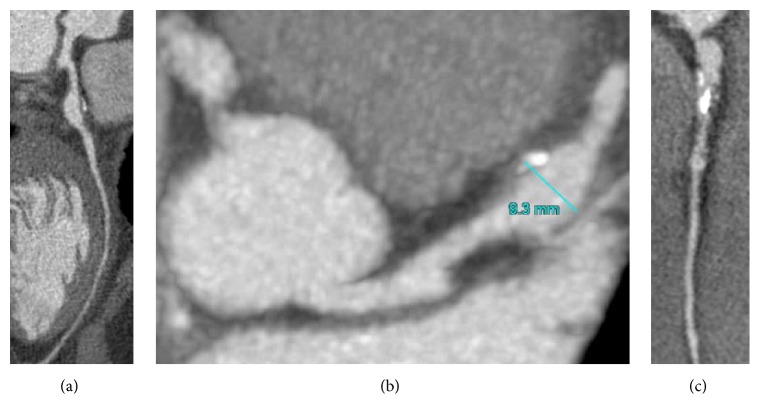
CT angiogram. (a) Curved multiplanar reformat image of the left anterior descending coronary artery. (b) Curved multiplanar reformat image of the right coronary artery. (c) Multiplanar reformat image demonstrating aneurysmal coronary arteries arising from aortic root.
